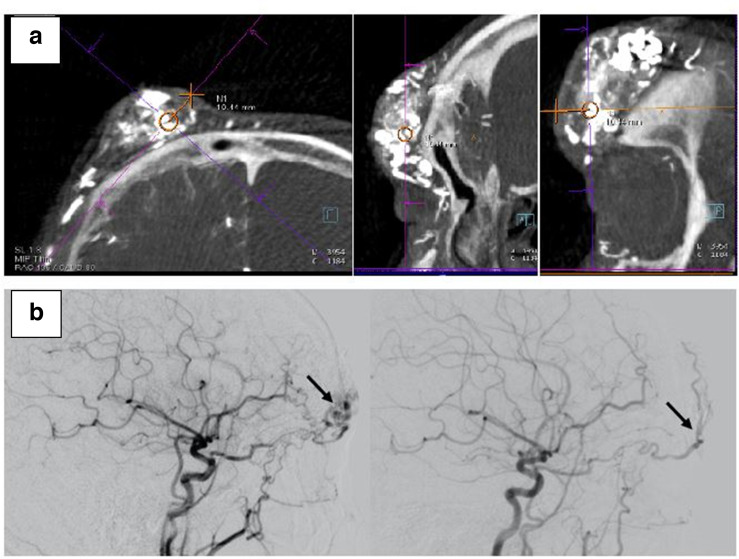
Case 1, AVM of the right frontal region. (a) CBCT-A in axial, sagittal, and coronal views demonstrating the projection of the virtual needle targeting the right supratrochlear artery. (b) DSA images showing (left) nidus at the left frontal region and (right) nidus occlusion following glue administration. AVM, arteriovenous malformation; CBCT-A, cone beam computed tomography angiography; DSA, digital subtraction angiography
